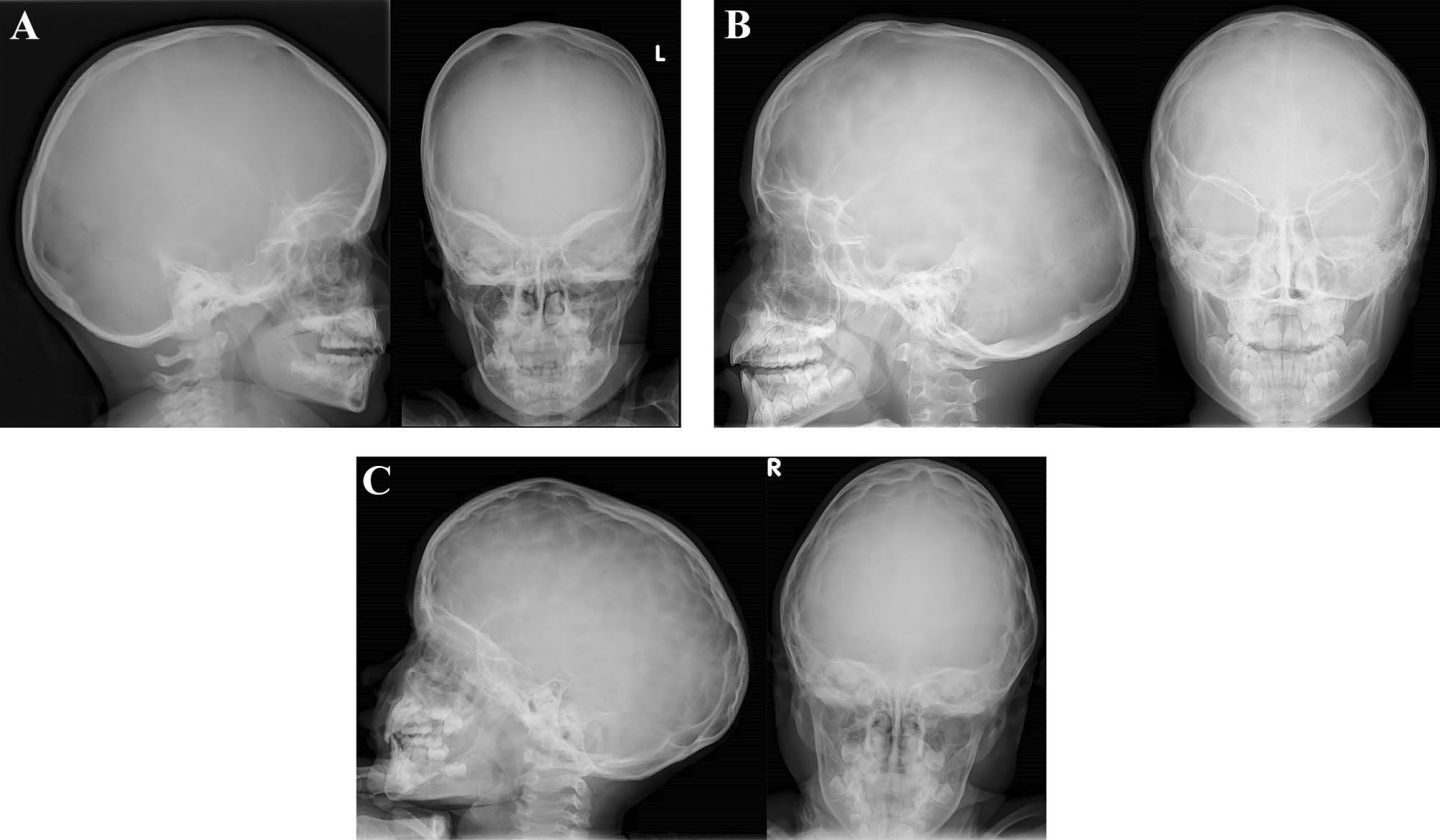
Fingerprinting on skull X-ray. A Normal skul X-ray, B minimal fingerprinting, C extensive fingerprinting
